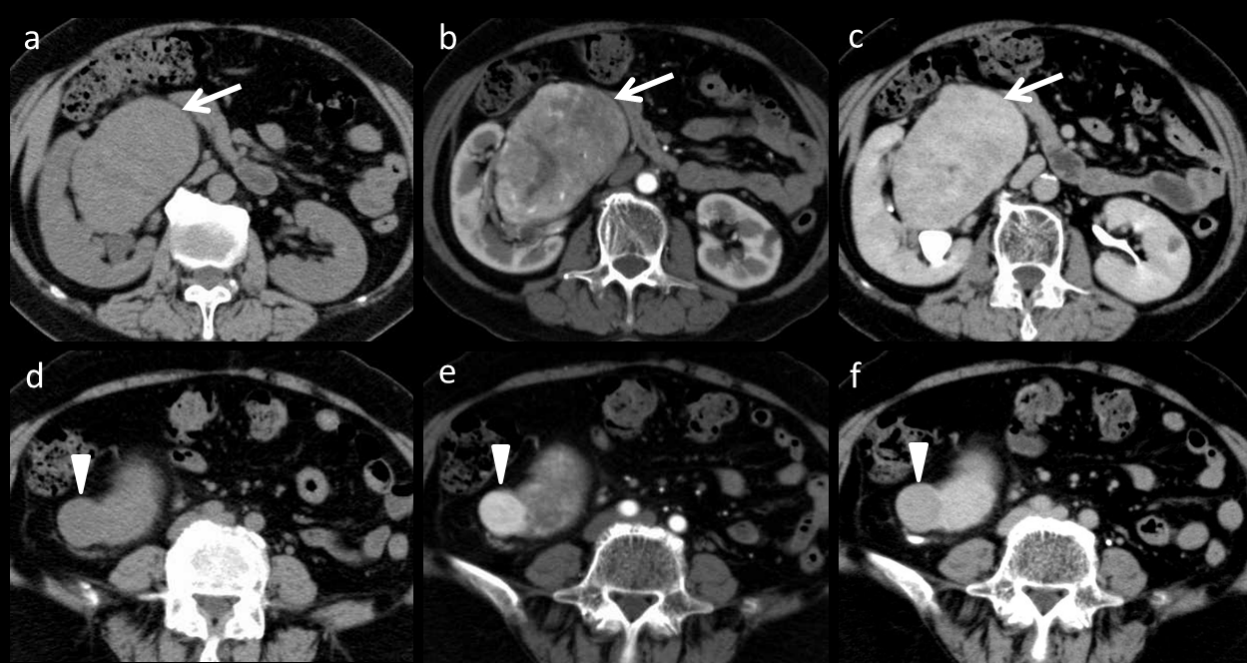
Axial non-contrast-enhanced CT image shows a large well-circumscribed lobulated tumor, arising from the hilum of the right kidney (a, d). The tumor consisted of two components with different patterns of enhancement. The corticomedullary (b) and nephrographic phases (c) showed moderate enhancement of the main part of the tumor. The small nodular component in the caudal portion of the tumor showed marked enhancement in the corticomedullary phase (e) and washout in the nephrographic phase (f)
